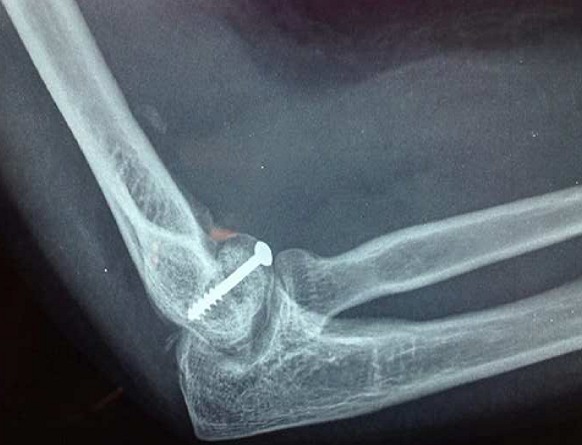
Contrôle radiographique de profil du montage
